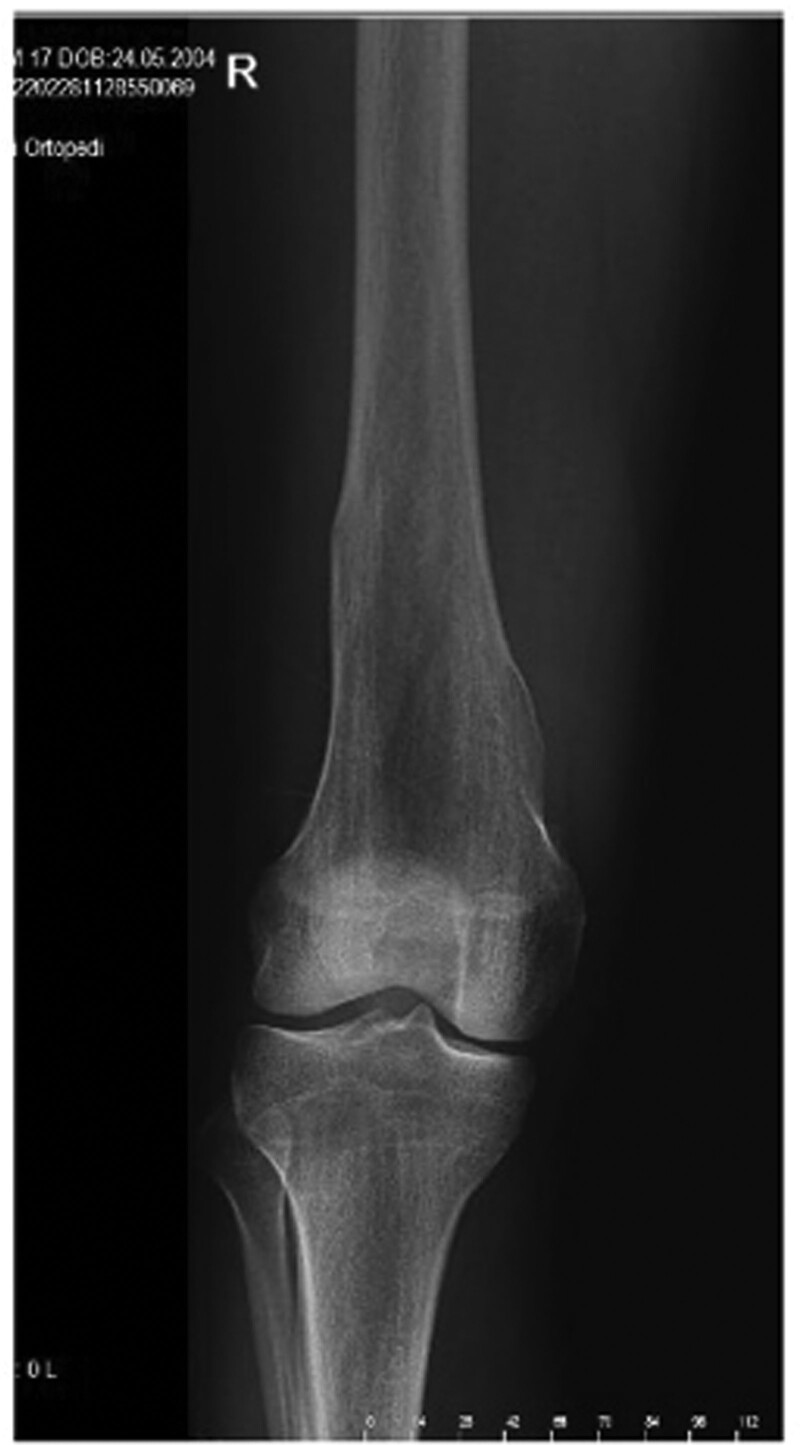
The X-ray of patient number 5 shows that their physis had closed, and all cartilage tissues had transformed into normal bone after 6 yr from the initial admission, at the age of 18 yr old. This is one of the clearest examples of transformation of the prominent cartilage column to the normal bone.
